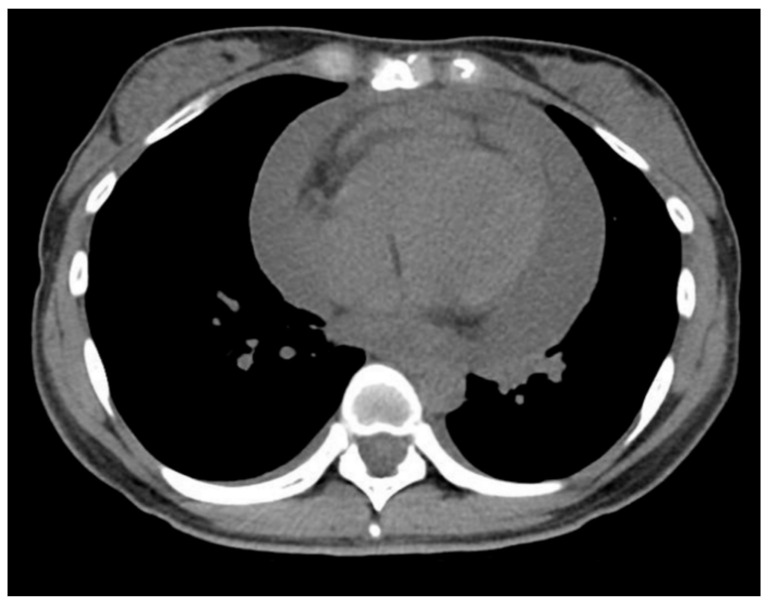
A computed tomography showing pericardial effusion.
